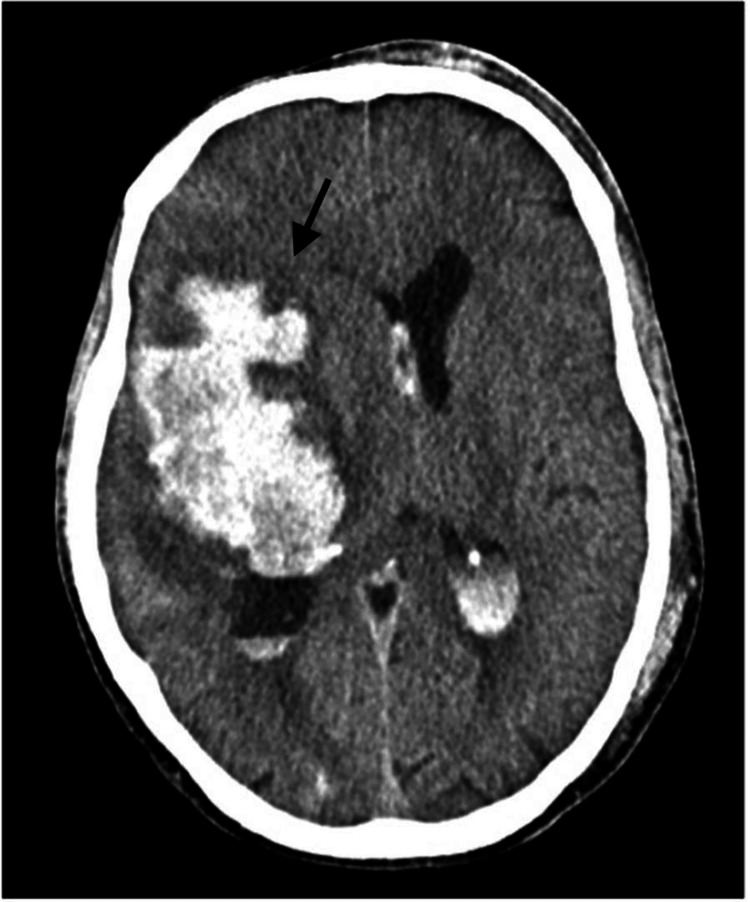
Brain computed tomography scan on admission showing right casual subarachnoid hemorrhage with intraparenchymal hemorrhagic lesion in the right temporal, insular, and frontal cortico-subcortical regions. Diffuse effacement of the cortical sulci in the right cerebral hemisphere, along with marked effacement of the right ventricle and a midline shift to the left of approximately 14 mm due to subfalcine herniation. There is also prominence of the left lateral ventricle, suggesting hydrocephalus, and intraventricular hemorrhage.
